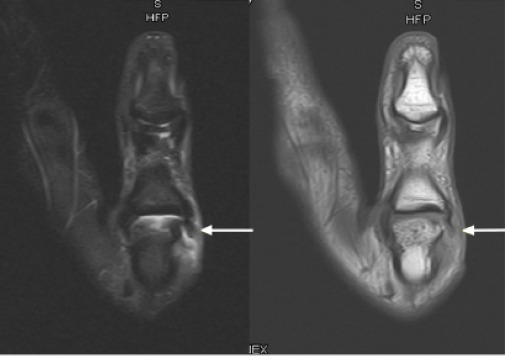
T1- (left) and T2- (right) weighted magnetic resonance imaging of the right thumb metacarpophalangeal joint demonstrating a complete tear of the proximal radial collateral ligament (white arrows).
